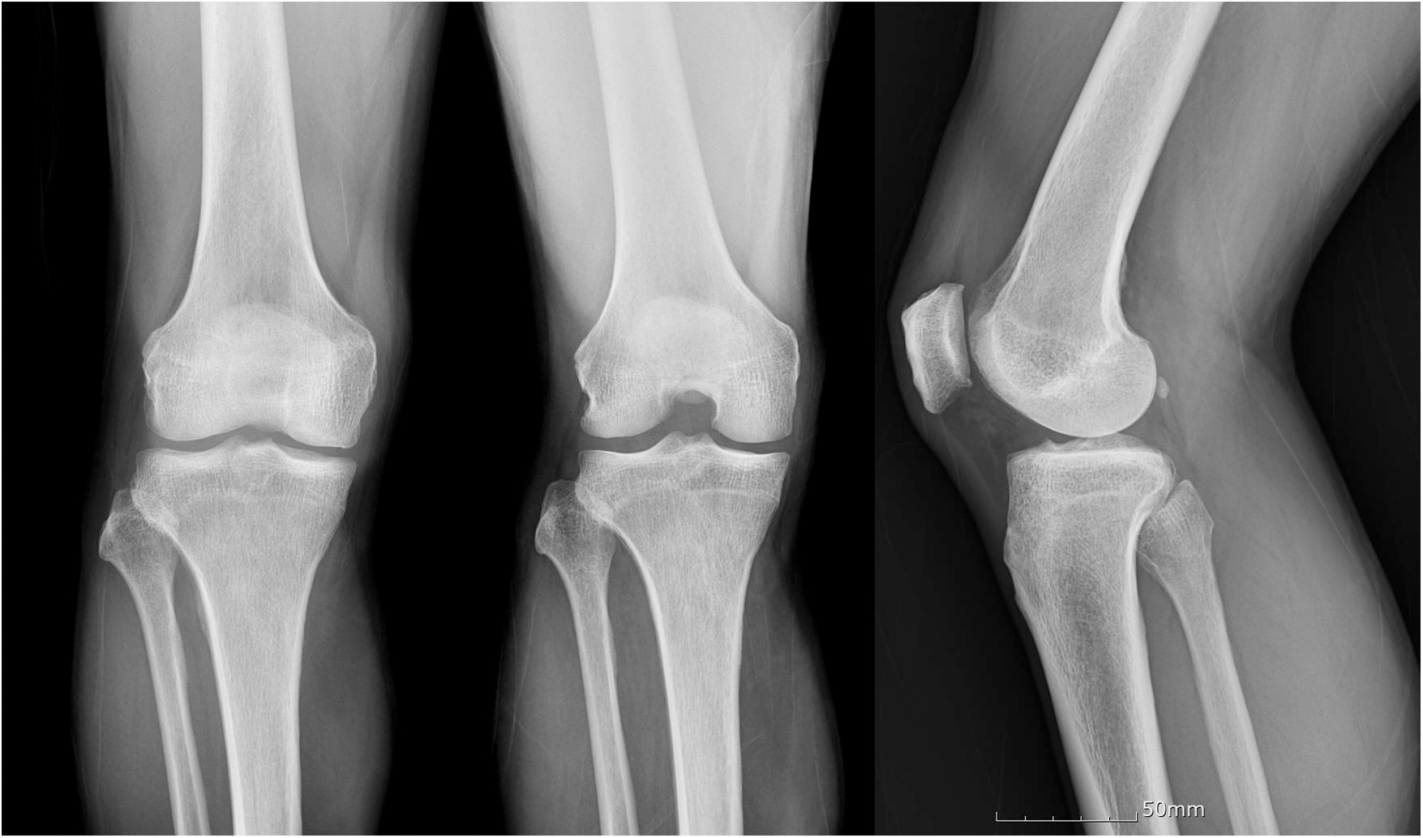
Preoperative standing anteroposterior, Rosenberg and lateral radiographs of the right knee showing mild degenerative changes and a narrowed intercondylar notch.
